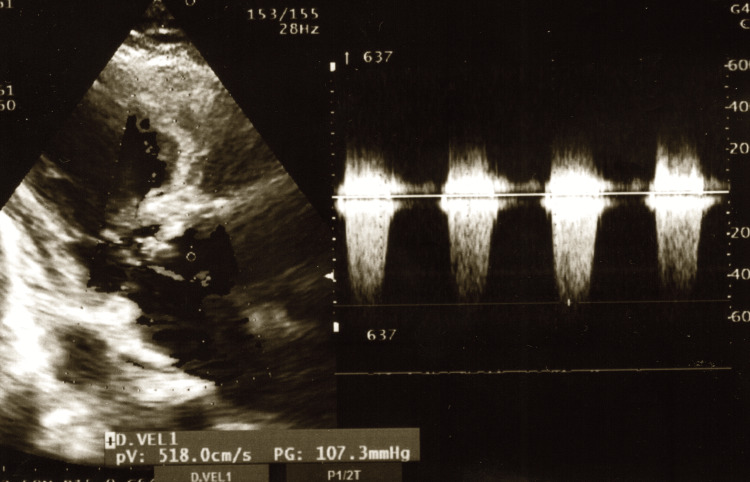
Echocardiography after installation of RV pacing This shows portable echocardiographic findings immediately after initiating temporary right ventricular (RV) pacing during cardiogenic shock. Color Doppler measurement revealed a left ventricular outflow tract pressure gradient of 107 mmHg, a marked improvement from 160 mmHg.
